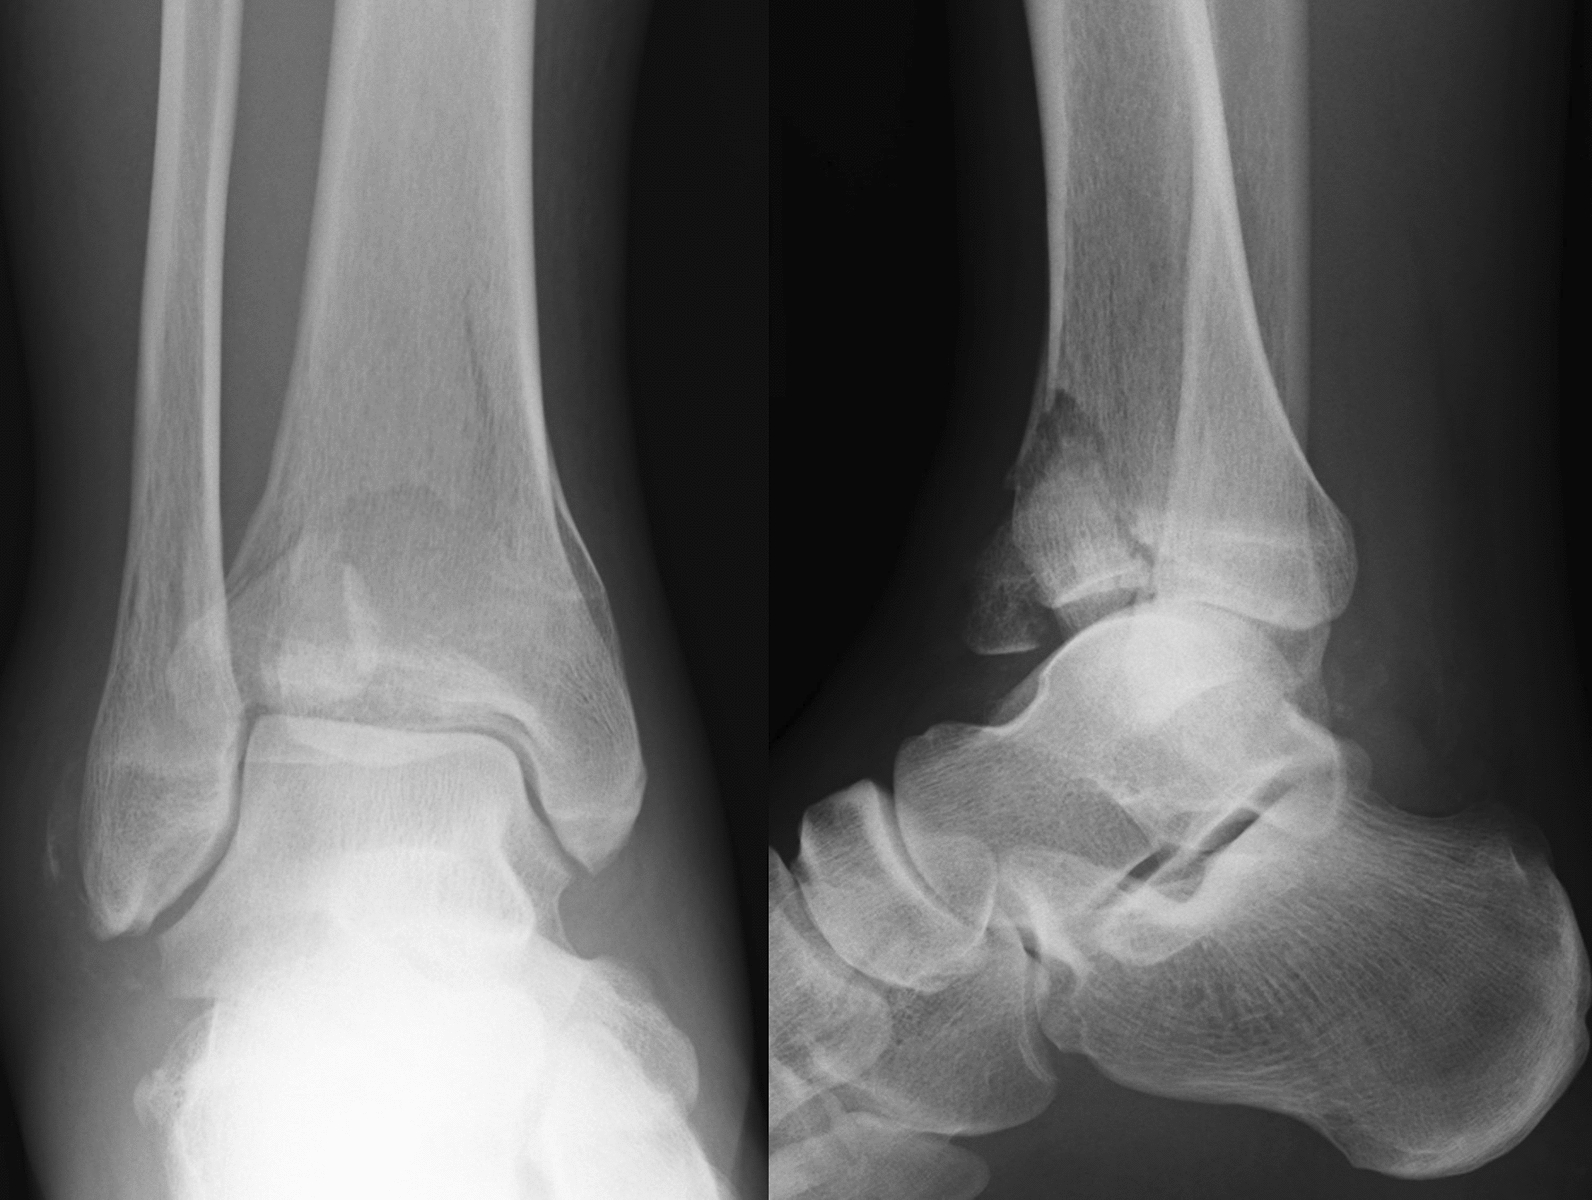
Plain radiographs of right ankle immediately after injury, showing a displaced pilon fracture: (right) anteroposterior view and (left) lateral view
